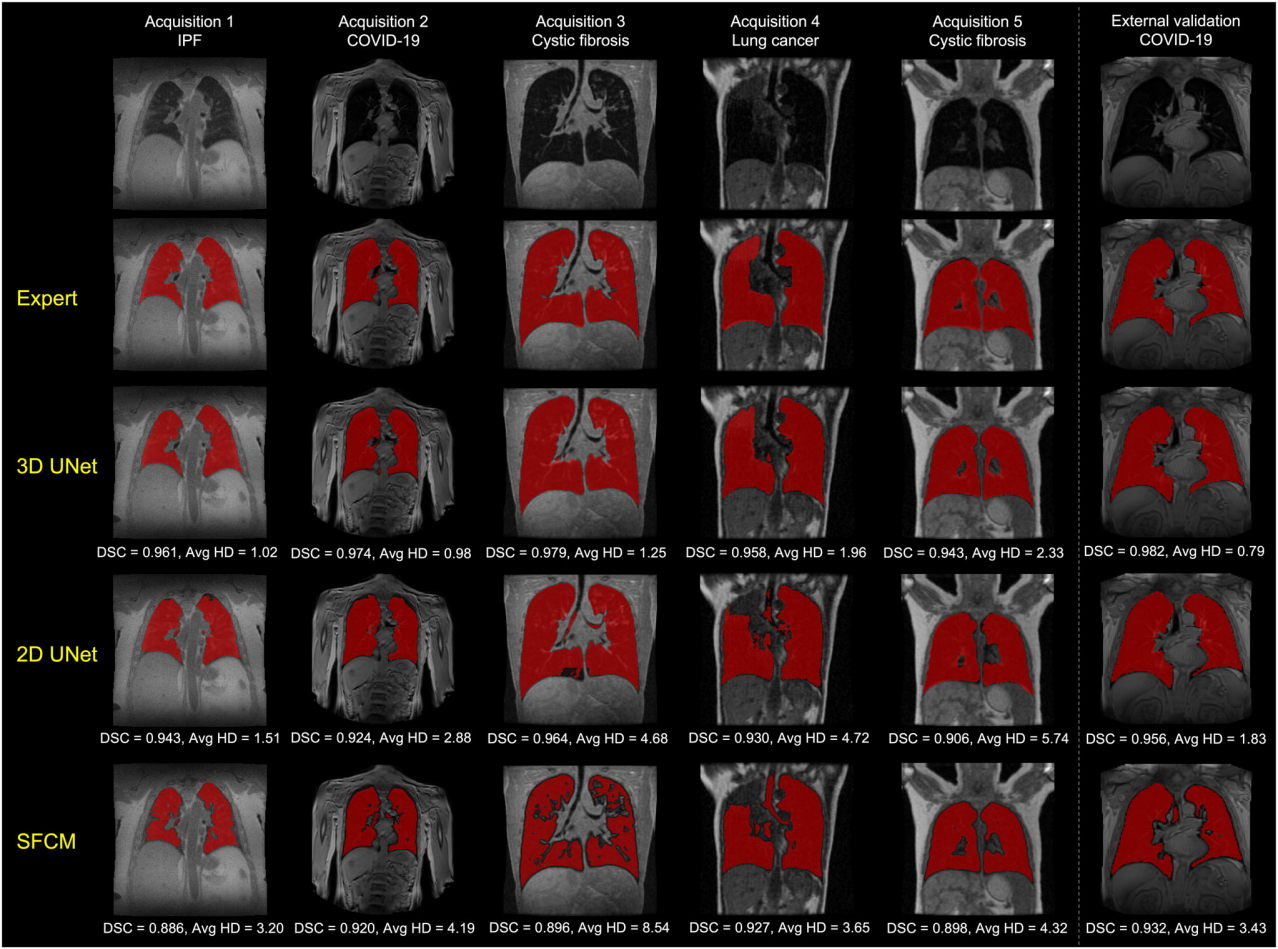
Example coronal slices showing the ^1^H‐MRI scans (row 1), the ^1^H‐MRI scans overlaid with manual segmentations (row 2) and segmentations generated by the 3D UNet, 2D UNet and spatial fuzzy c‐means (SFCM) methods (rows 3–5) for six representative cases. Dice similarity coefficient (DSC) and average Hausdorff distance (HD) values are provided for each case. Example slices were left uncropped to display differences in field of view and arm position between acquisitions.
